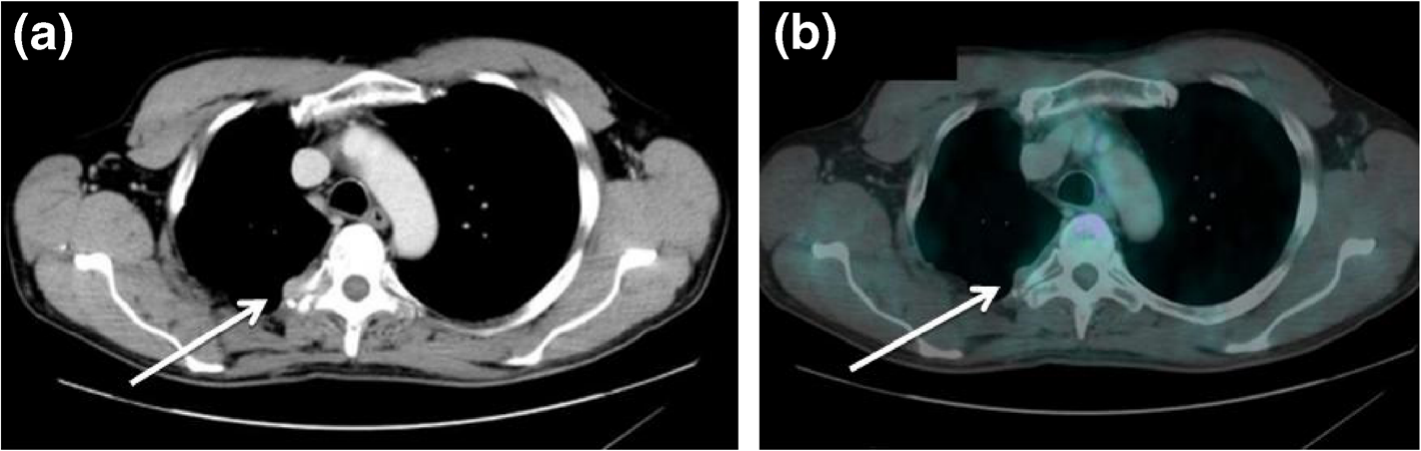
A 51-year-old male patient, presenting tumor marker increase after surgery for lung cancer. (a) Axial, contrast-enhanced, full-dose CT showed a paravertebral lesion, which was slightly enlarged and significantly more hyperemic with respect to a previous CT scan. (b) The lesion showed very mild FDG uptake; it was, however, classified as highly suspicious on the basis of the contrast-enhanced CT component of the exam. Biopsy confirmed the presence of lung adenocarcinoma metastasis. Accordingly, the patient was referred for radiotherapy. CT, computed tomography; FDG, [^18^ F] fluorodeoxyglucose.
